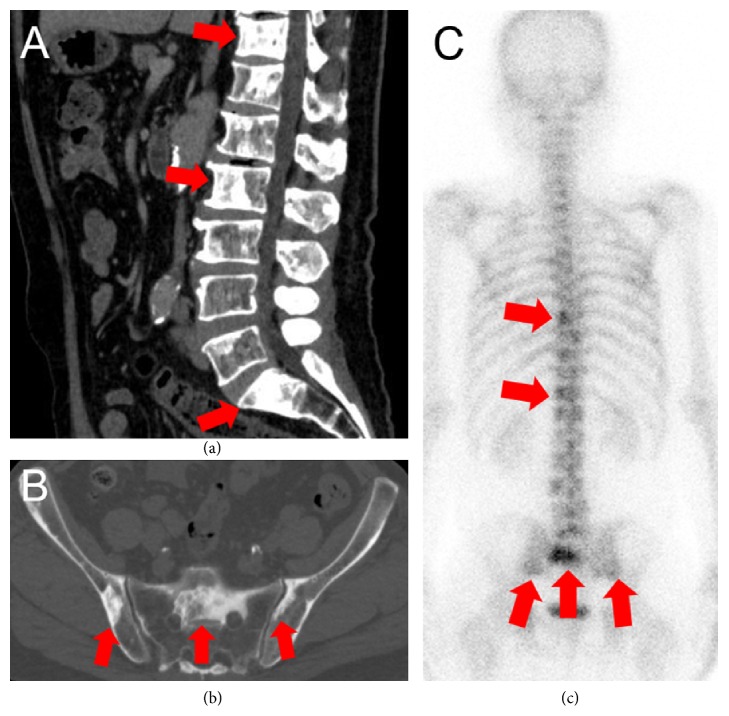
Imaging findings at the time of CRPC diagnosis. CT showed multiple osteoblastic lesions in the spine (a) and pelvic bone (b). Bone scan confirmed multiple accumulations at the same bone sites as CT (c). CRPC, castration-resistant prostate cancer; CT, computed tomography.
